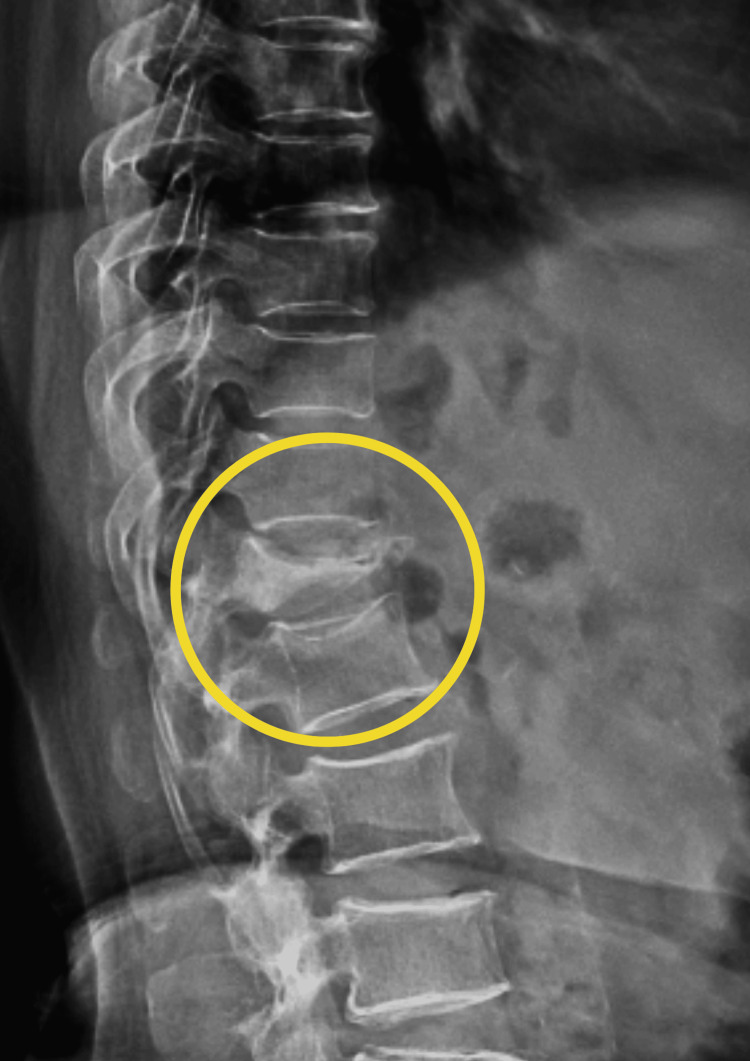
Pre-operative X-ray of the spine The yellow arrow shows a D12 vertebra wedge compression fracture.
